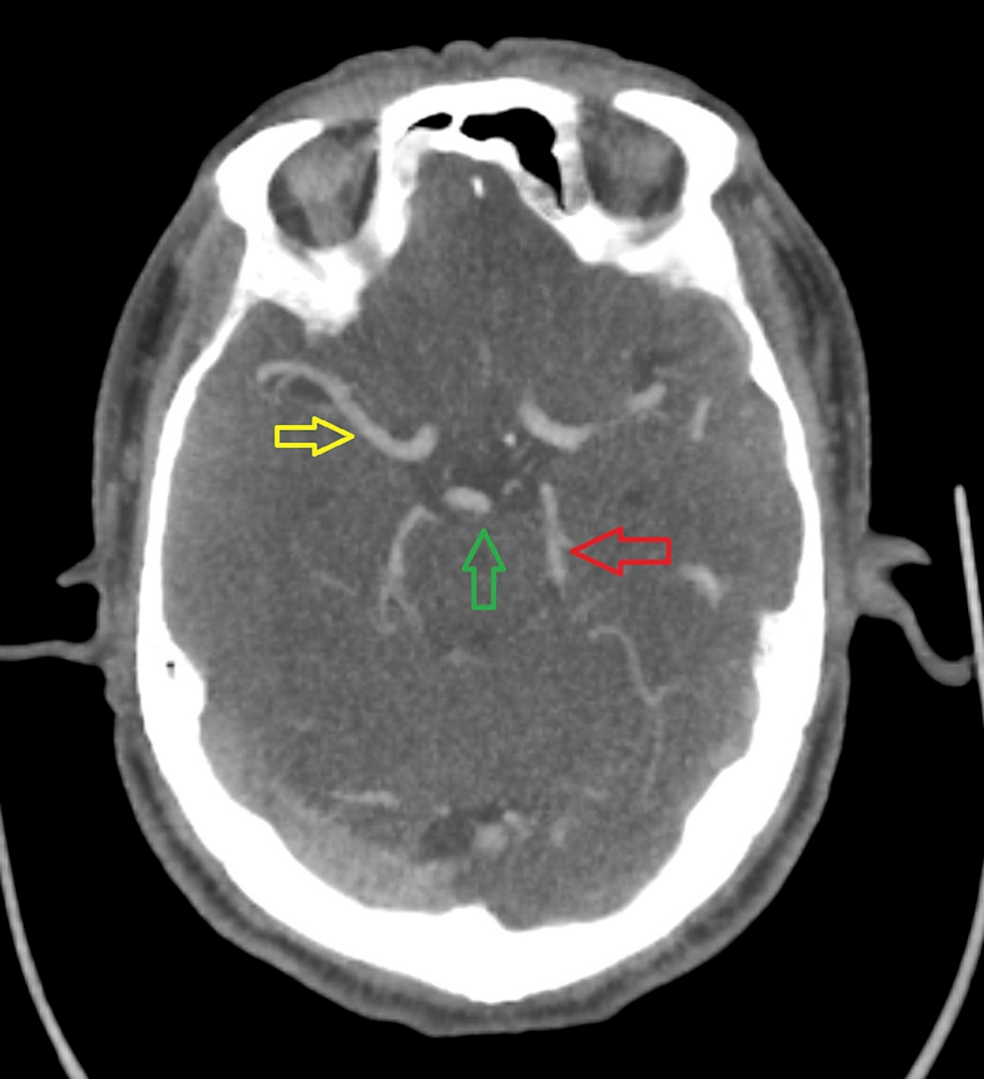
CTA of the brain showed patent anterior and posterior circulation. The yellow arrow points to the right middle cerebral artery. The red arrow points to the left posterior cerebral artery. The green arrow points to the tip of the basilar artery.
